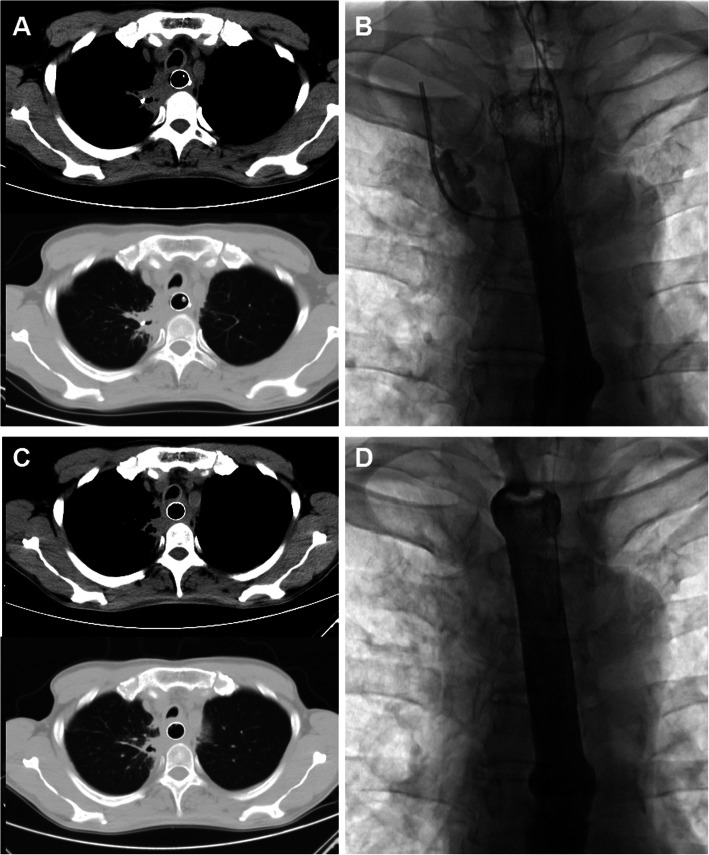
Examinations after stent placement during follow up. a The chest CT scan shows decrease of mediastinal abscess with no pleural effusion 2 month after procedure. b Esophagography shows decrease of esophageal mediastinal abscess, and then the drainage tube was removed 2 months after procedure. c–d The chest CT scan and esophagography shows almost disappearance of mediastinal abscess 2.7 months after stent placement
